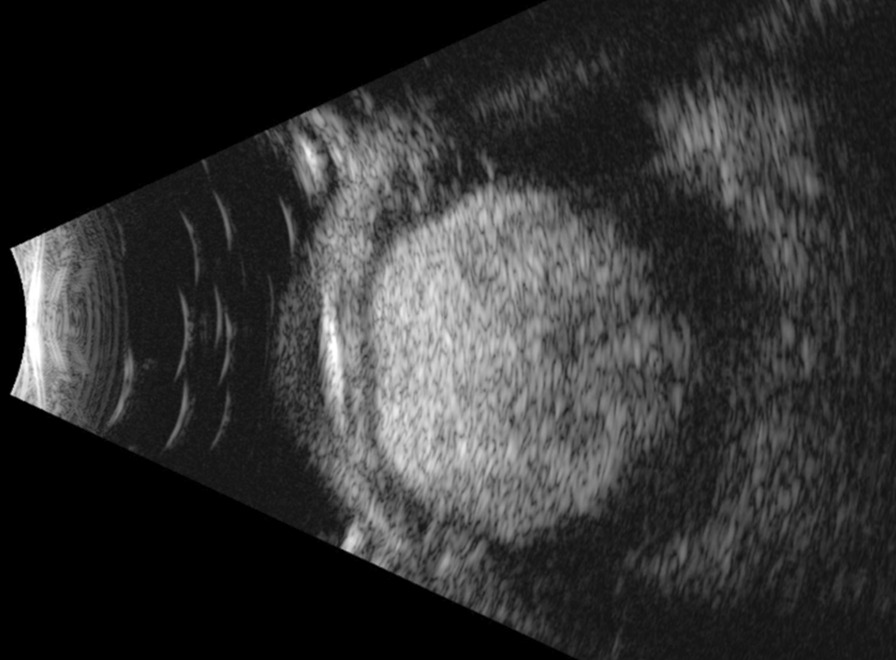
10 MHz immersion B-scan ultrasound displaying a large ciliary body lesion in its entirety
